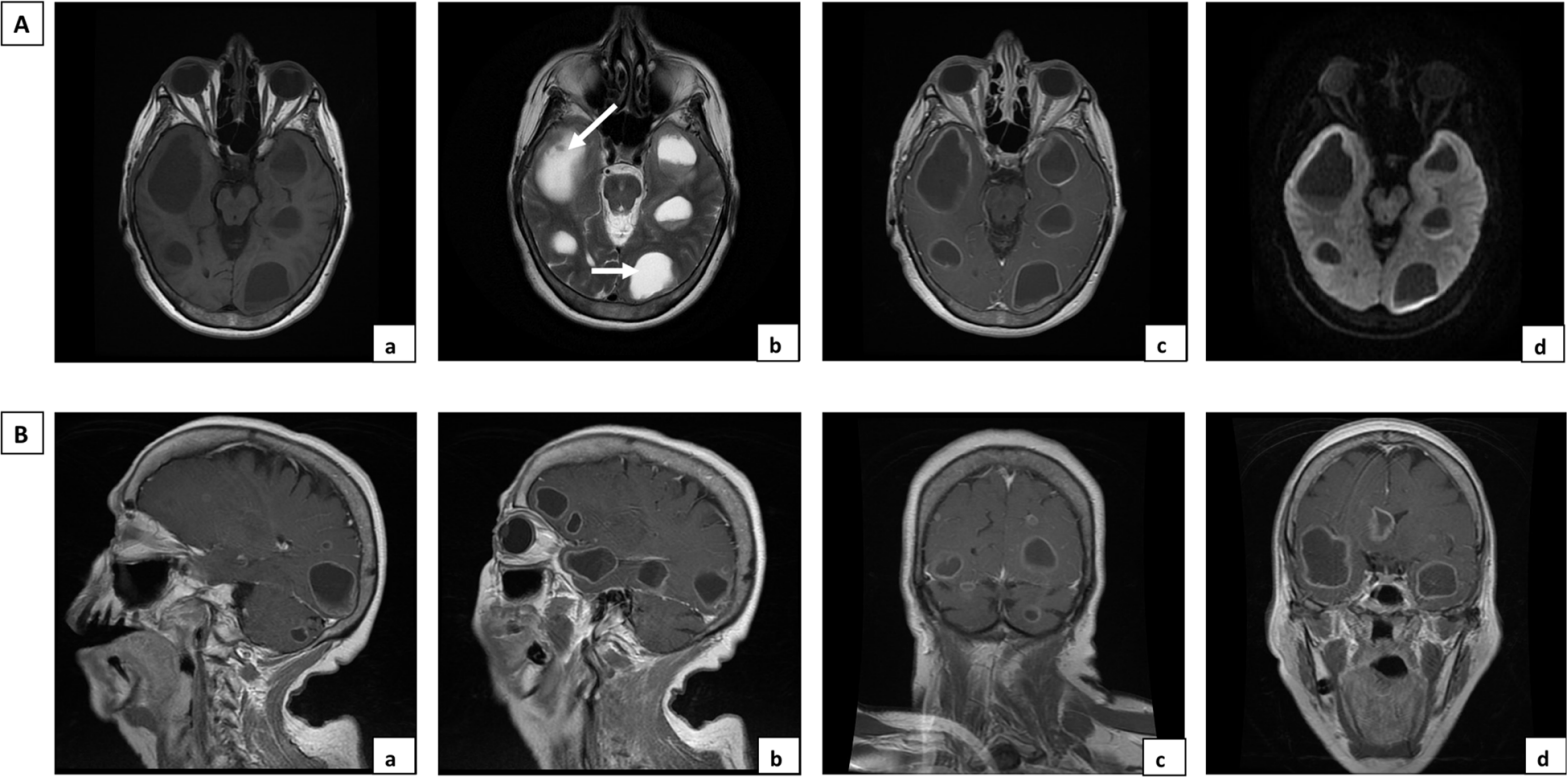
Cranial nuclear magnetic resonance image. A. Multiple cystic lesions with ring enhancement after contrast administration, without restricted diffusion, in temporal and occipital lobes. (a) Axial T1SE, (b) Axial T2 Propeller, (c) Axial T1 SE + gadolinium, (d) Axial DWI. B. Gadolinium contrast brain NMRI showing ring-enhanced lesions with multilobar distribution. (a) and (b) Sagittal T1 SE + gadolinium, (c) coronal T1 SE + gadolinium with supra- and infratentorial lesions, (d) coronal T1 SE + gadolinium with temporal and intraventricular lesions
